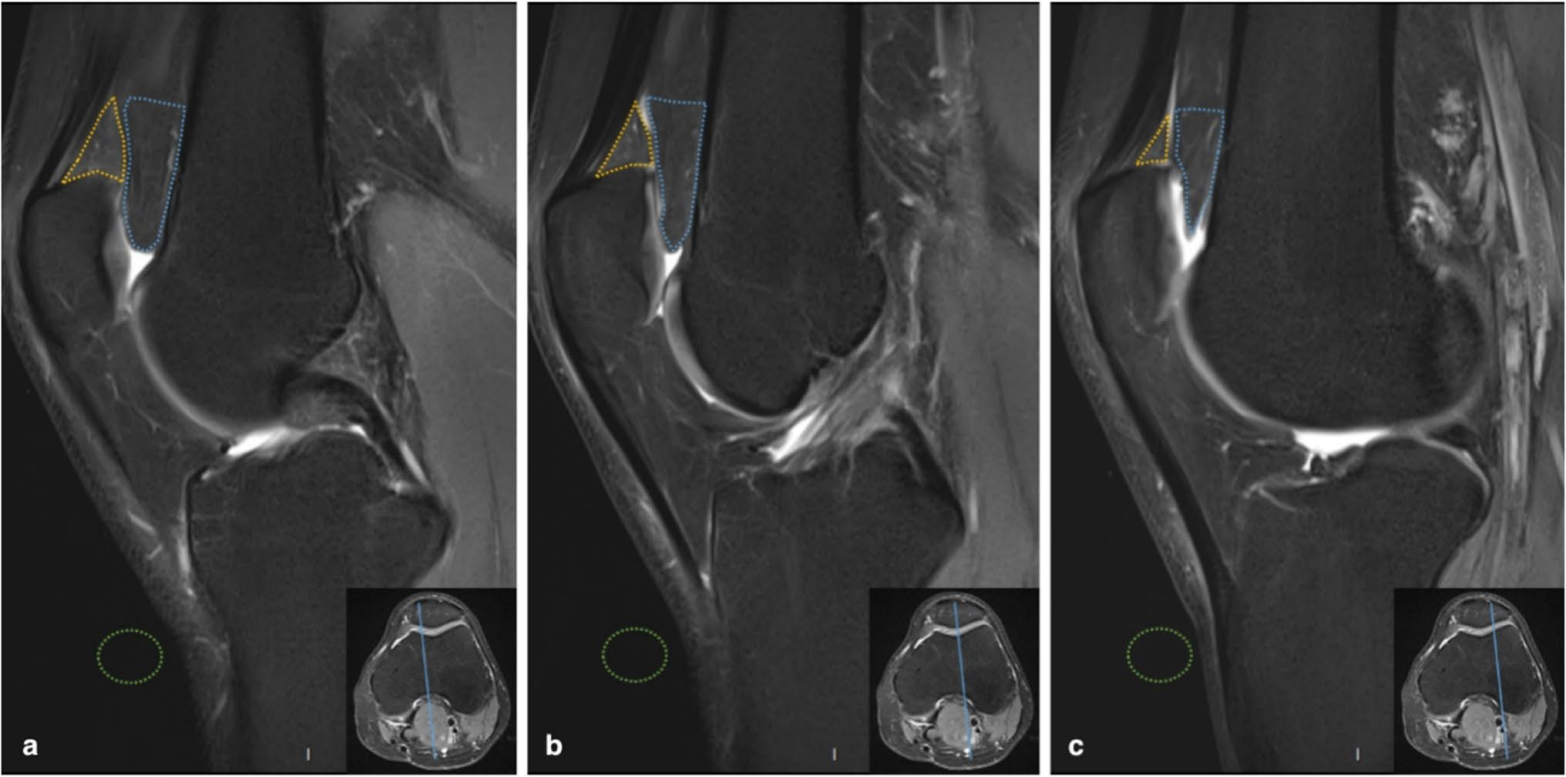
MRI 3 T sagittal PD TSE fat-suppressed slices. SPFP relative signal intensity was measured in three slices at the midpoint of the medial trochlear facet (a), at the center of the trochlea (b), and at the midpoint of the lateral trochlear facet (c). For each slice, three regions of interest (ROIs) were drawn: one around the SPFP (yellow), one around the prefemoral fat pad (blue) at the same level, and one in the air (green) in the lower part of the image for the background noise
